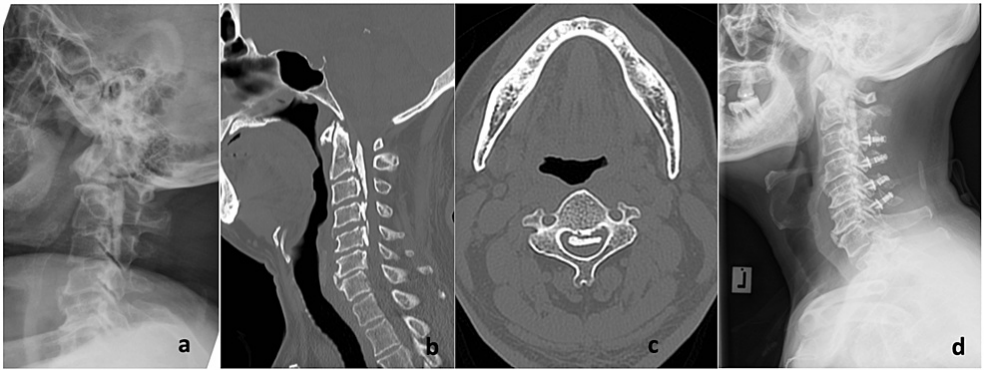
Patient with multiple-level cervical OPLL a: Plain radiograph showing the presence of cervical lordosis; b: Presence of multiple mixed-type cervical OPLL from C2 to C5; c: Occupying ratio is less than 60%; d: Laminoplasty was performed using a plate system OPLL: Ossification of the posterior longitudinal ligament
